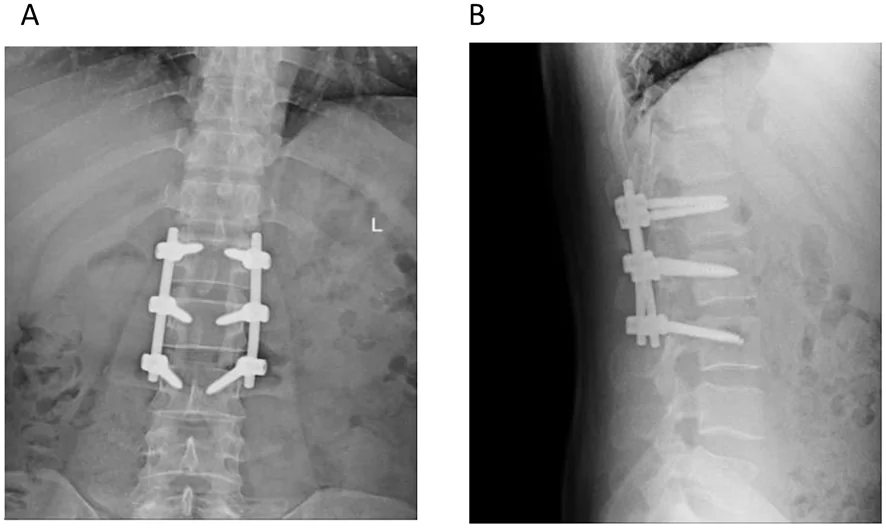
Radiographic follow-up findings at two weeks postoperatively (A, B) Anteroposterior and lateral lumbar spine radiographs demonstrate preserved natural lumbar lordosis and orderly vertebral alignment; mild osteophytic changes are observed in several vertebral bodies and associated posterior elements; no significant spinal canal stenosis is present.
